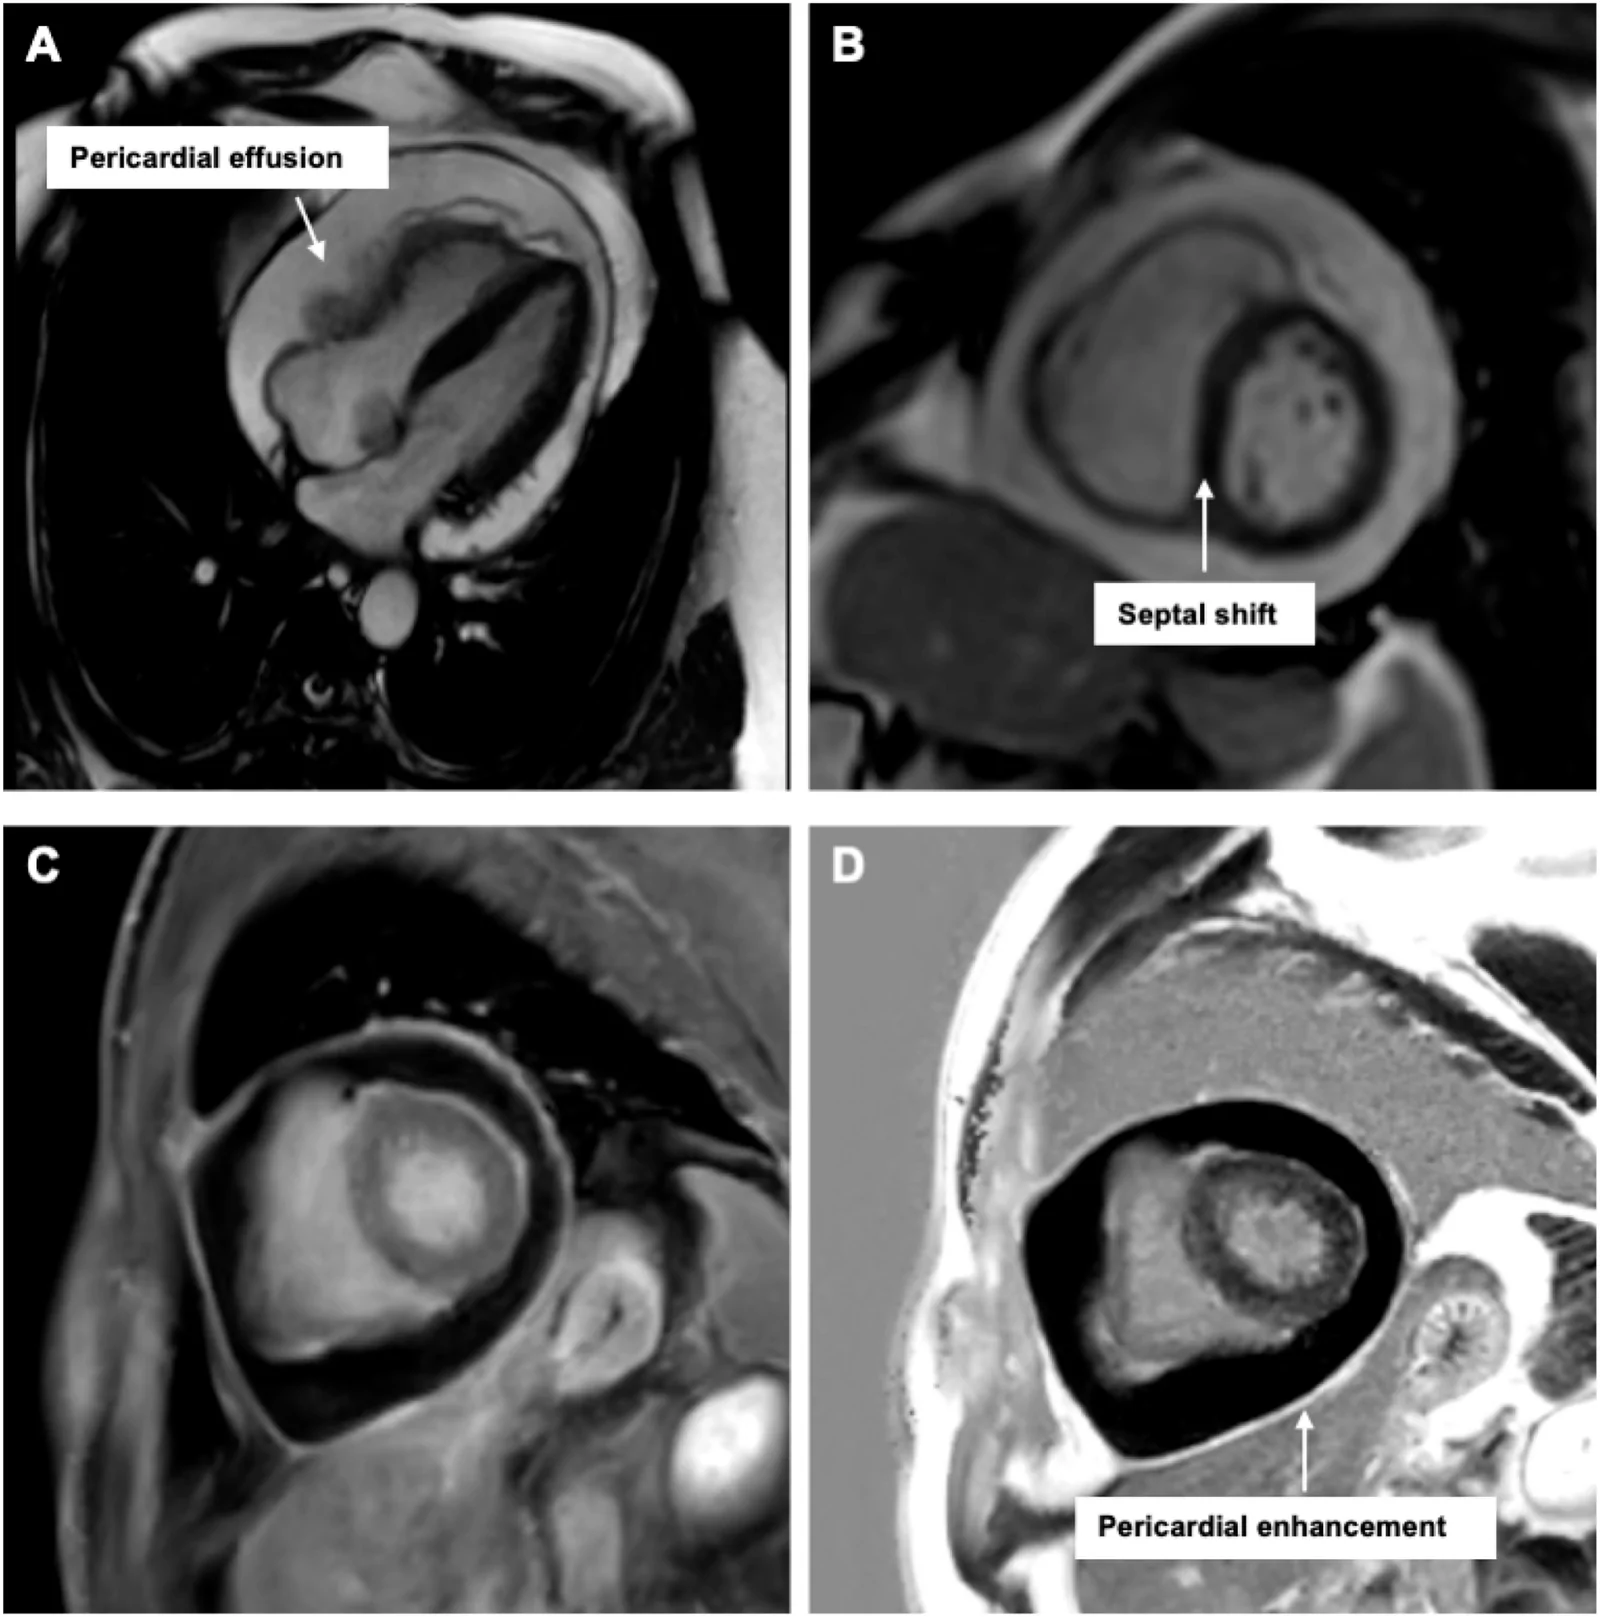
Cardiac magnetic resonance imaging demonstrating constrictive pericarditis. (A) Four-chamber cine image showing circumferential pericardial effusion. (B) Short-axis cine image demonstrating septal shift consistent with constrictive physiology. (C) T2-weighted short-axis image showing no myocardial edema. (D) Late gadolinium enhancement short-axis image demonstrating circumferential pericardial enhancement without myocardial enhancement.
